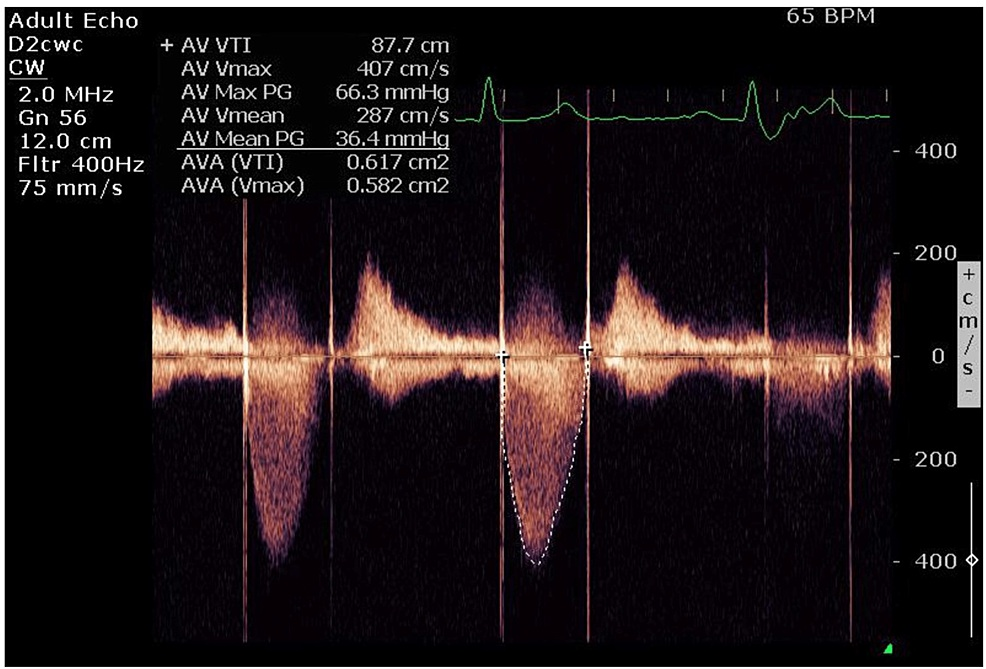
Repeat TTE post first cycle of tPA therapy showed a mean transvalvular gradient of 36 mmHg and peak transvalvular gradient of 66 mmHg. TTE, transthoracic echocardiogram; tPA, tissue plasminogen activator
